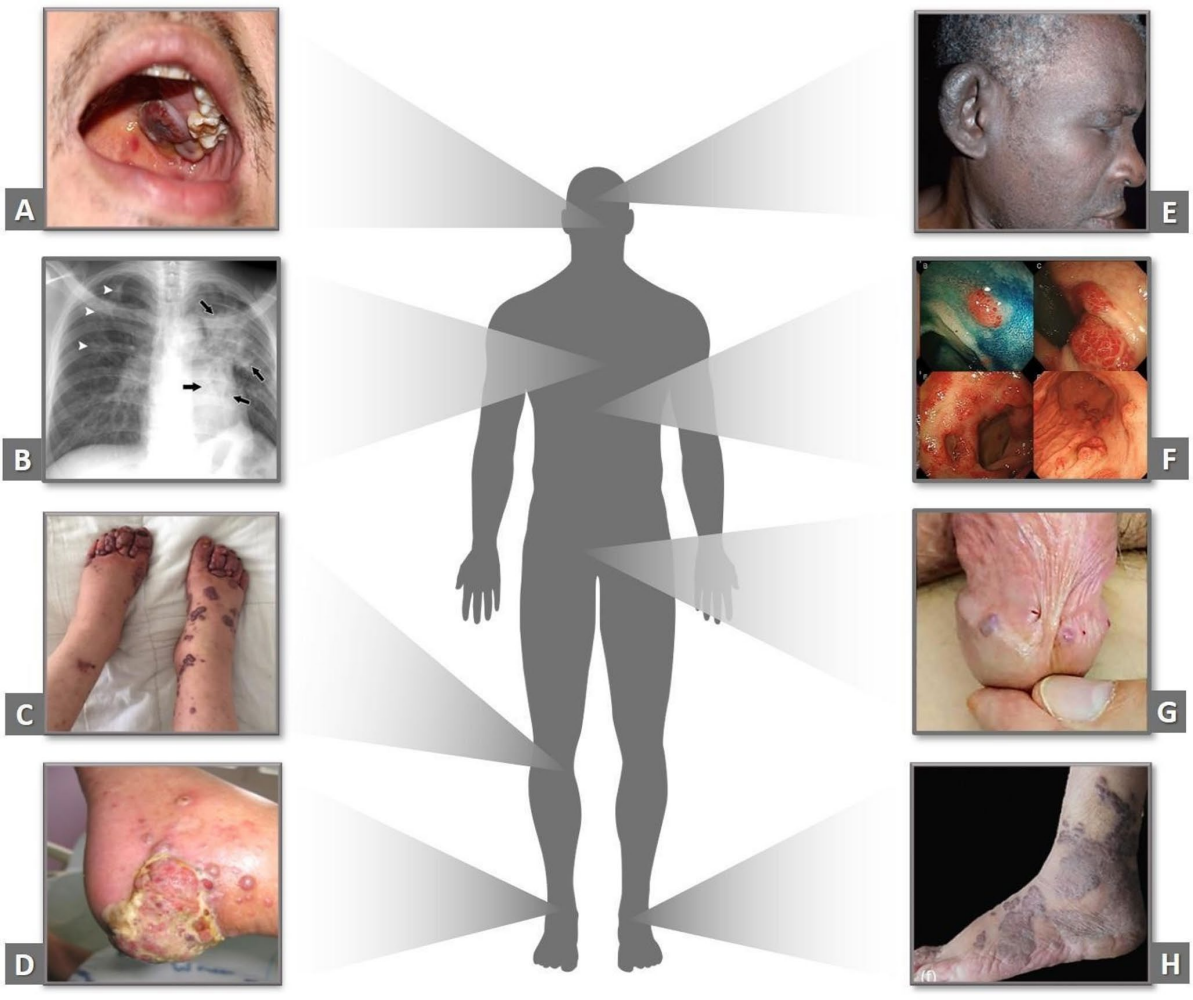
Clinical manifestations of Kaposi’s sarcoma across different anatomical sites and epidemiological forms. (A) Oral Kaposi’s sarcoma in an HIV-positive patient, showing violaceous nodular and plaque lesions on the hard palate. (B) Pulmonary involvement in an HIV-positive patient with KS, detected by chest X-ray, showing bilateral nodular and mass-like lesions (arrows). (C) Angiomatous cutaneous nodules associated with lymphedema in a patient with IKS. (D) Advanced cutaneous KS with tumor expansion and surrounding nodules in the heel of a patient with AKS. (E) Diffuse tumor infiltration of the external ear in a patient with AEKS. (F) Visceral involvement of KS-HIV patient, demonstrated by endoscopic images of gastrointestinal tract lesions. (G) Genital involvement in CKS, presenting as erythematous–violaceous papules on the glans penis. (H) Multiple violaceous cutaneous nodules of varying sizes affecting the forefoot in CKS. Images reproduced from published sources as indicated in panels A–H:Ma J.Y. and Liu J.W., 2022 (A); Restreo C.S.Martinez S. et al., 2006 (B); Elboukhari K., Achehboune K. et al. 2020 (C); Chapalain M., Goldman-Lévy G. et al. 2018 (D); Travassos A.R., Costa J.B. et al. 2010 (E); Nagata N., Shimbo T. et al., 2012 (F); Ramos M.C., Oliveira F.B. et al., 2020 (G); Zhou J. Shen X.Wang, X. et al. 2021 (H).
